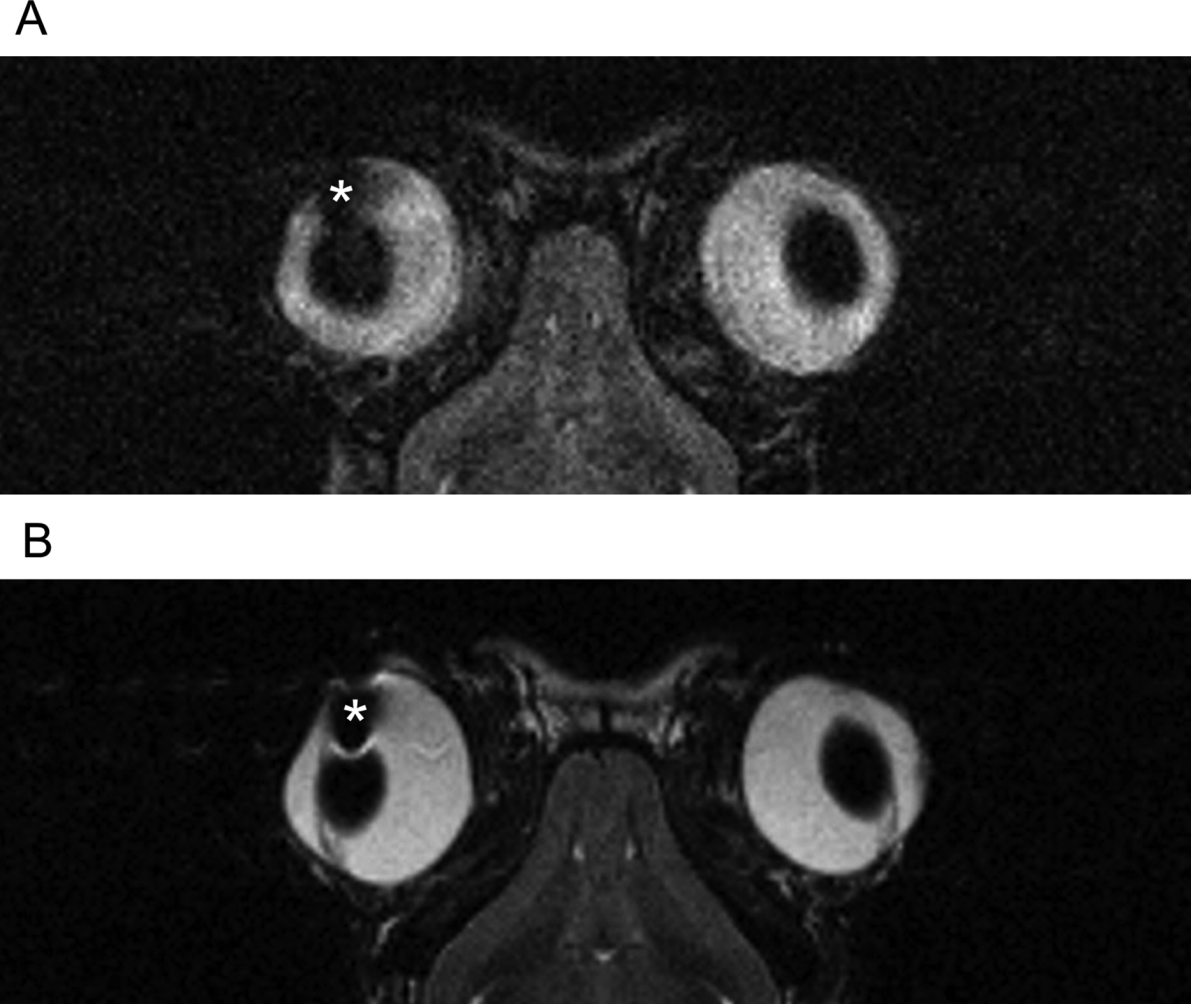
T2-weighted MR images of the Ex-PRESS-inserted eyes. (A) Image under low-SAR conditions. (B) Image under high-SAR conditions. * The Ex-PRESS insertion site. MR, magnetic resonance.
